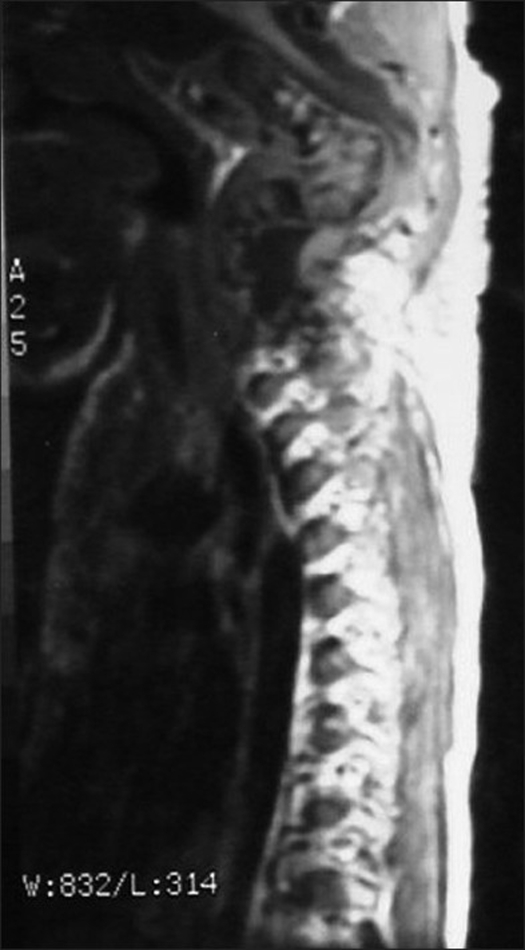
The magnetic resonance imaging (T_2_W sagital view) shows the stretched out cervical cord that was compressed at the apex of the kyphosis
